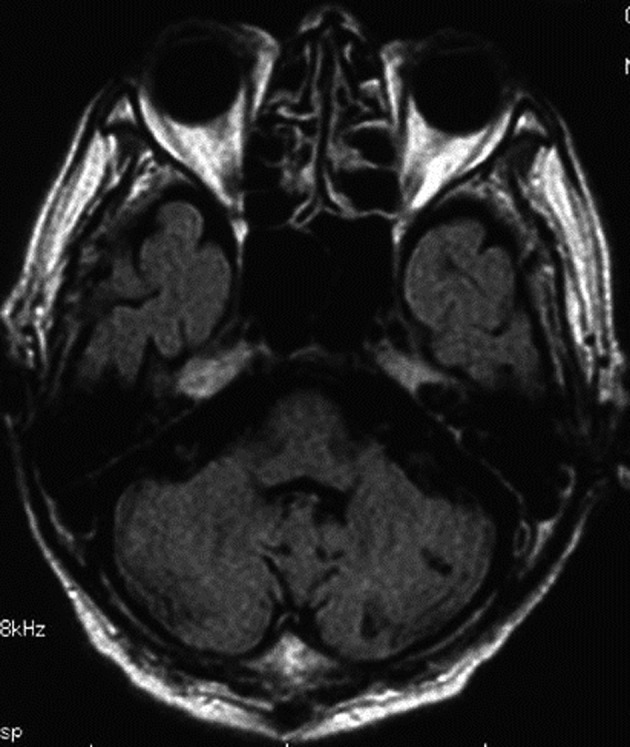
The hyperintensity of the dendric nuclei in MRI was disappeared one month later.
